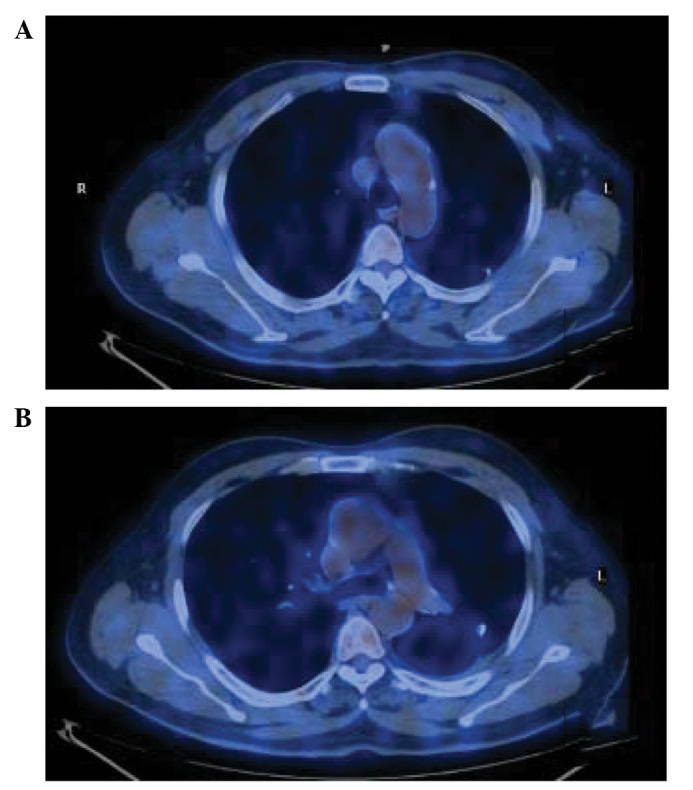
Follow-up of ^18^F-fluorodeoxyglucose positron emission tomography/computed tomography (^18^F-FDG-PET/CT) (December 3, 2011). (A) Nodules in the ascending aorta/aortic arch wall have disappeared (normal ^18^F-FDG uptake), (B) as has the nodule in the pericardial wall (normal ^18^F-FDG uptake).
